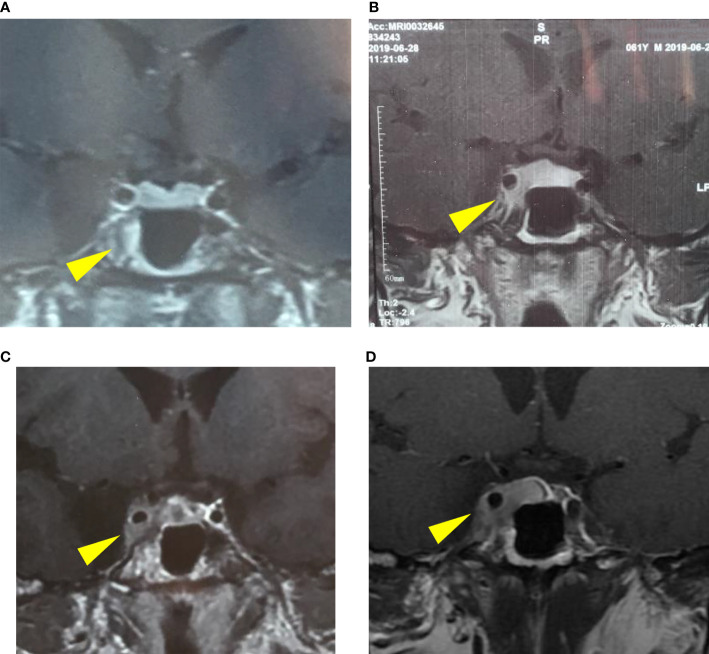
The progression of the pituitary lesion in MRI: (A) a suspicious low signal in the right-wing of the pituitary (January 15, 2019), (B) enlargement of the pituitary lesion involving right cavernous sinus (June 28, 2019), (C) involvement of the right cavernous sinus and internal carotid artery (September 24, 2019), (D) a possible macroadenoma, Knosp IV (October 17, 2019).
